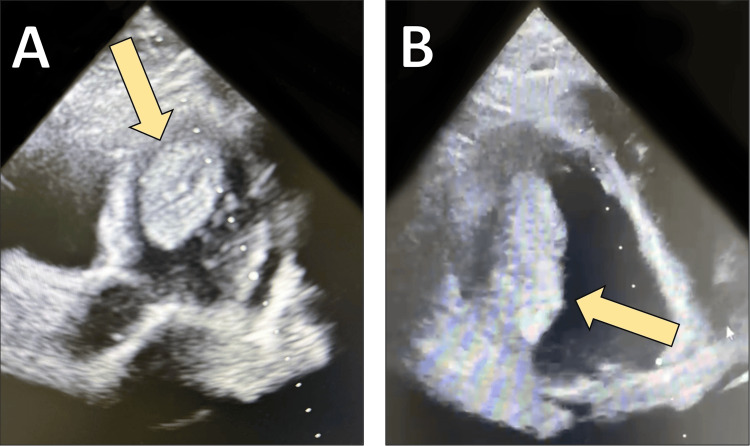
Transthoracic echocardiography demonstrating left ventricular thrombus A: four-chamber view revealing a large thrombus in the apical region of the left ventricle (yellow arrow); B: short-axis view confirming the presence and size of the apical thrombus (yellow arrow).
